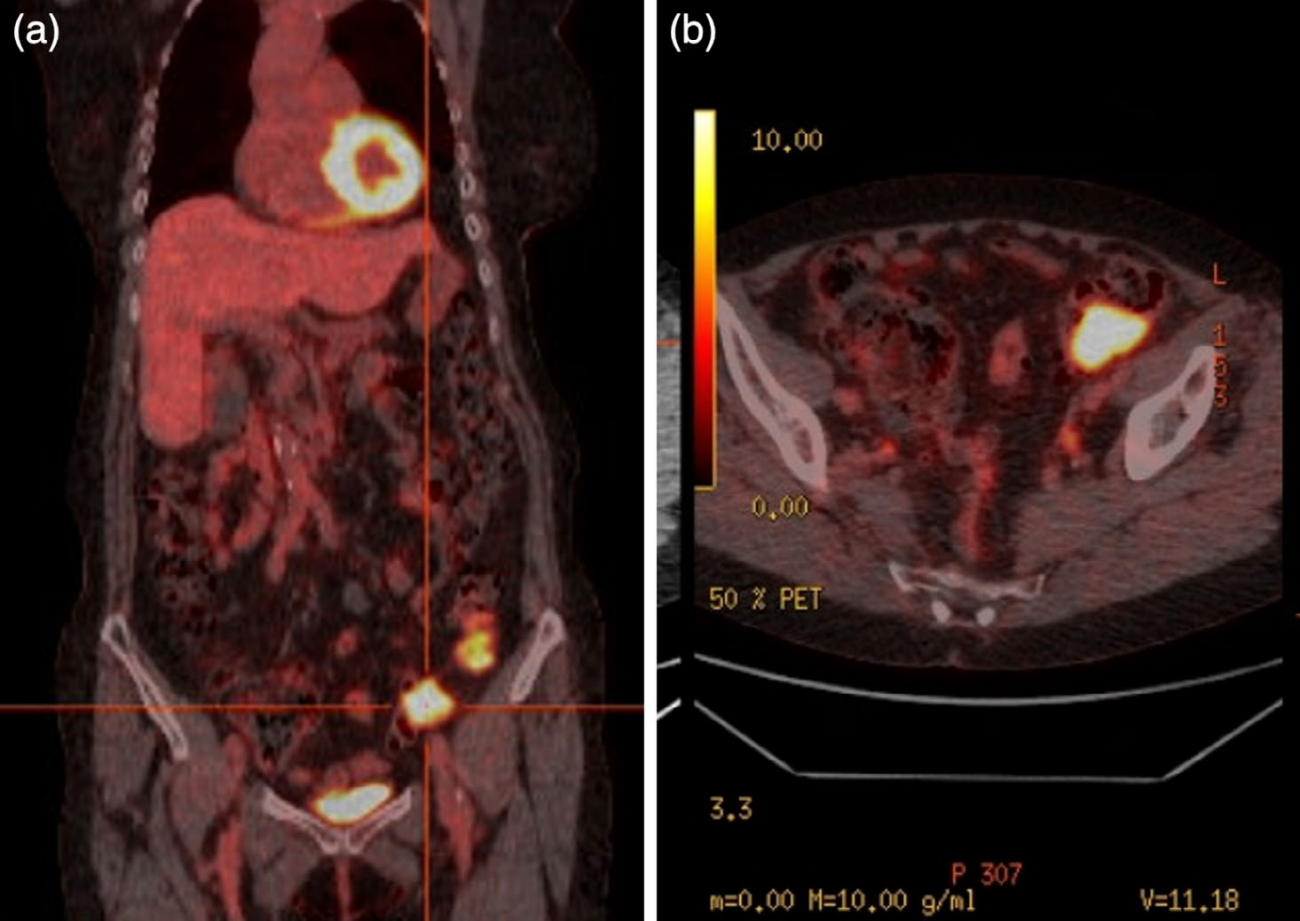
PET Whole Body FDG Study. (a) Coronal view showing two foci of FDG uptake. The crosshairs indicate the location of the soft tissue sigmoid lesion. (b) Axial view showing intense FDG uptake in the soft tissue sigmoid lesion.
